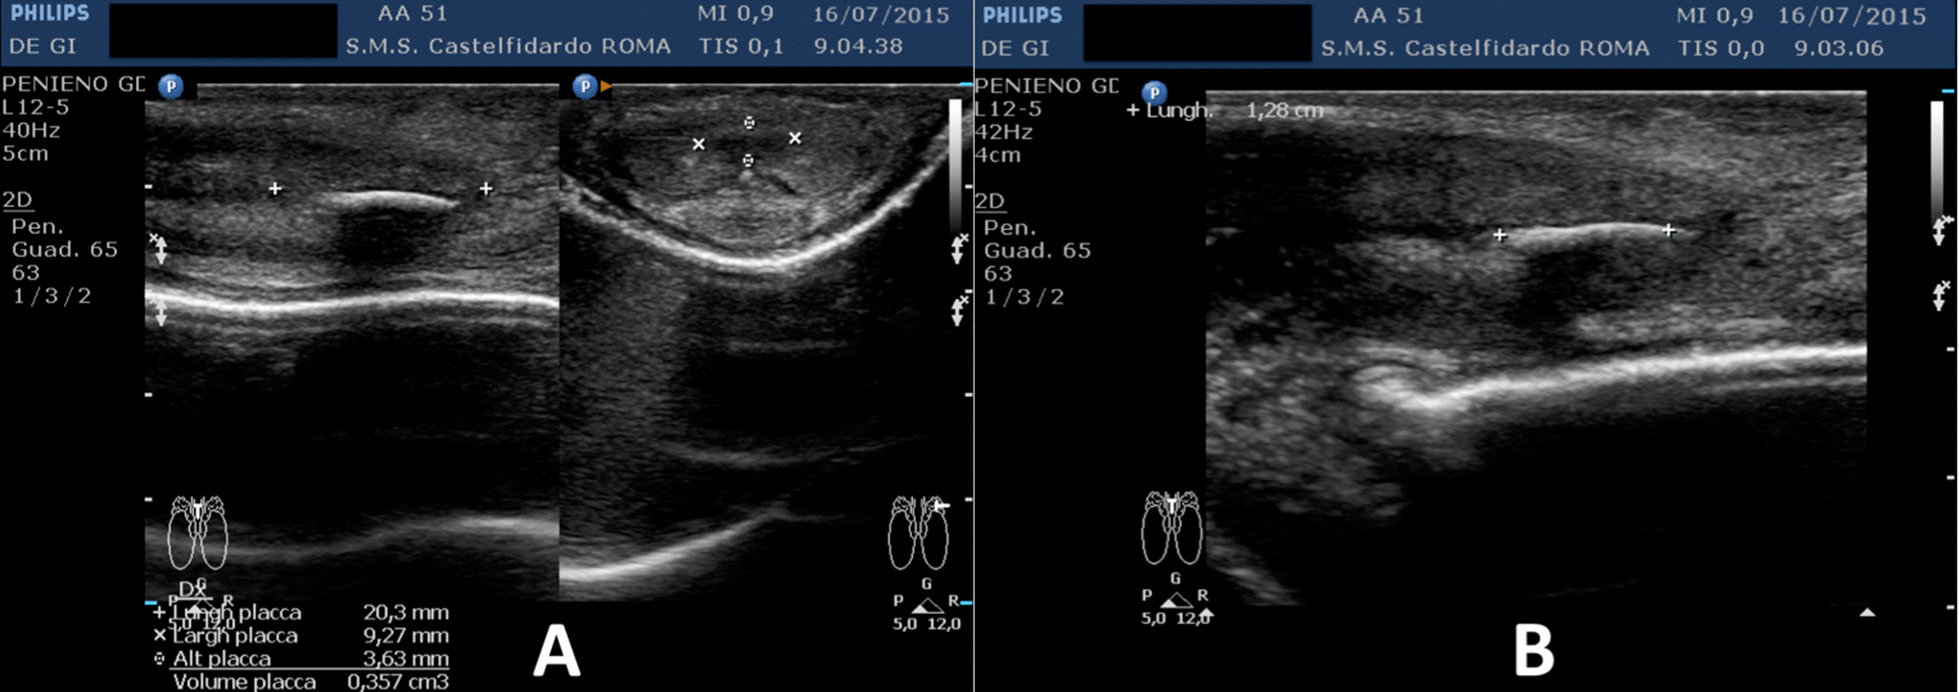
Penile ultrasound after the first treatment cycle (12 months). A Plaque measurement in longitudinal and transverse scan. B Calcification measurement in longitudinal scan
